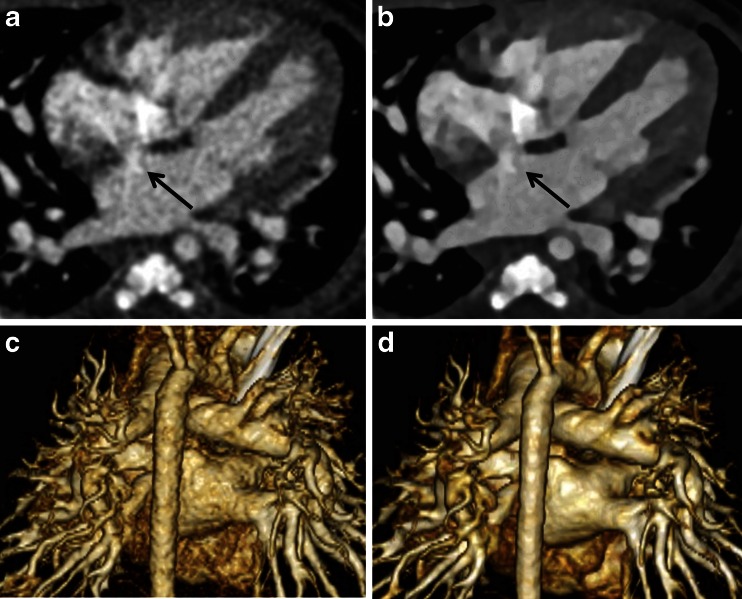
A 1-month-old girl with a ventricular septal defect and atrium septal defect (*arrows* in a and b). CT was scanned using the 70-kV protocol. a-b Transverse images are reconstructed by a conventional filtered back projection algorithm (S0) and sinogram-affirmed iterative reconstruction with the 5th strength (S5), respectively. c-d Volume-rendered images reconstructed by S0 and S5, respectively
